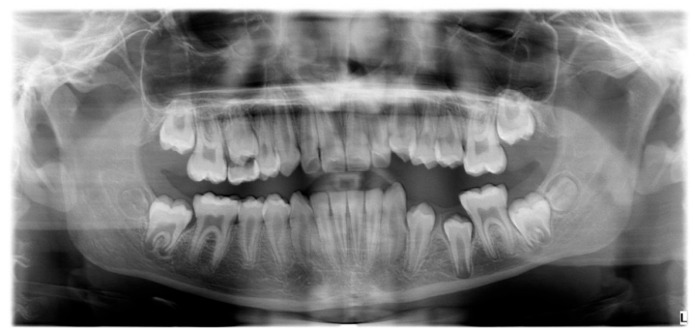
Orthopantomography X-ray after the treatment (clinical case #2).
